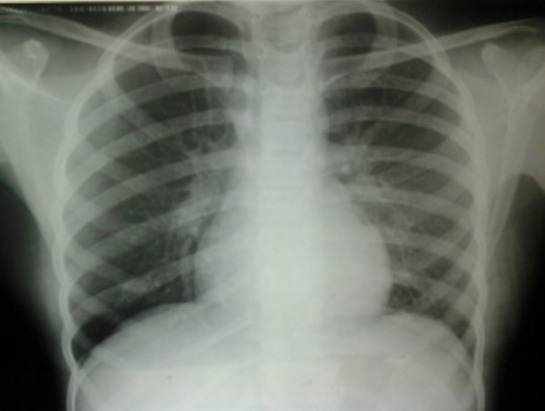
Radiographie thoracique: opacités hilaires
